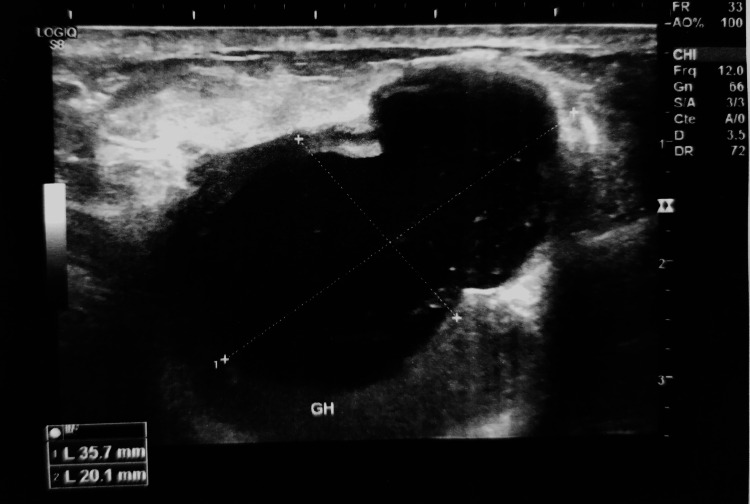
Sonographic appearance of the tumor. Hypoechoic lesion in the junction of upper quadrant with irregular contours measuring 35 mm x 20 mm.
